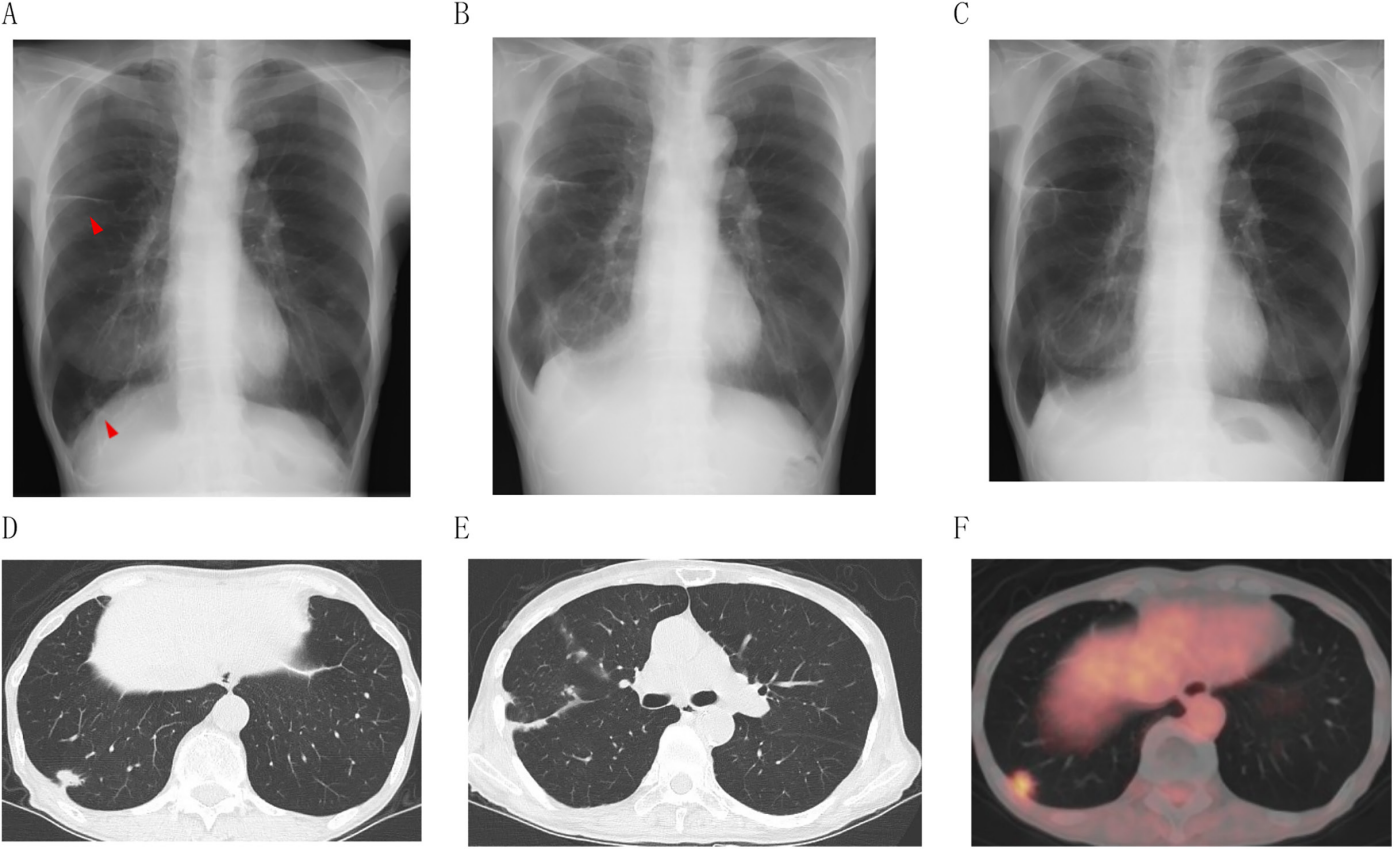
(A–C) Chest radiograph before surgery revealed thickening of the right minor fissure and a right lower lung field nodule (A), which had progressed before the start of selpercatinib (B), but improved 2 weeks after starting selpercatinib (C). (D, E) Chest computed tomography (CT) showed a nodule 17 mm in size in the right lower lobe (D) and multiple small nodules along the right interlobar pleura (E), suggesting pleural dissemination. (F) ^18^F‐FDG positron emission tomography (PET)‐CT showed elevated FDG uptakes in the right lower lobe nodule and multiple nodules on the interlobar pleura.
